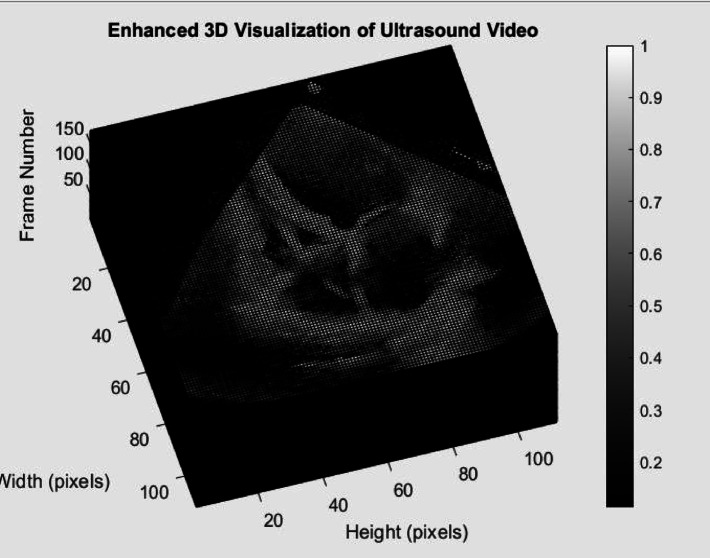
3D view of the ultrasound image of heart.
